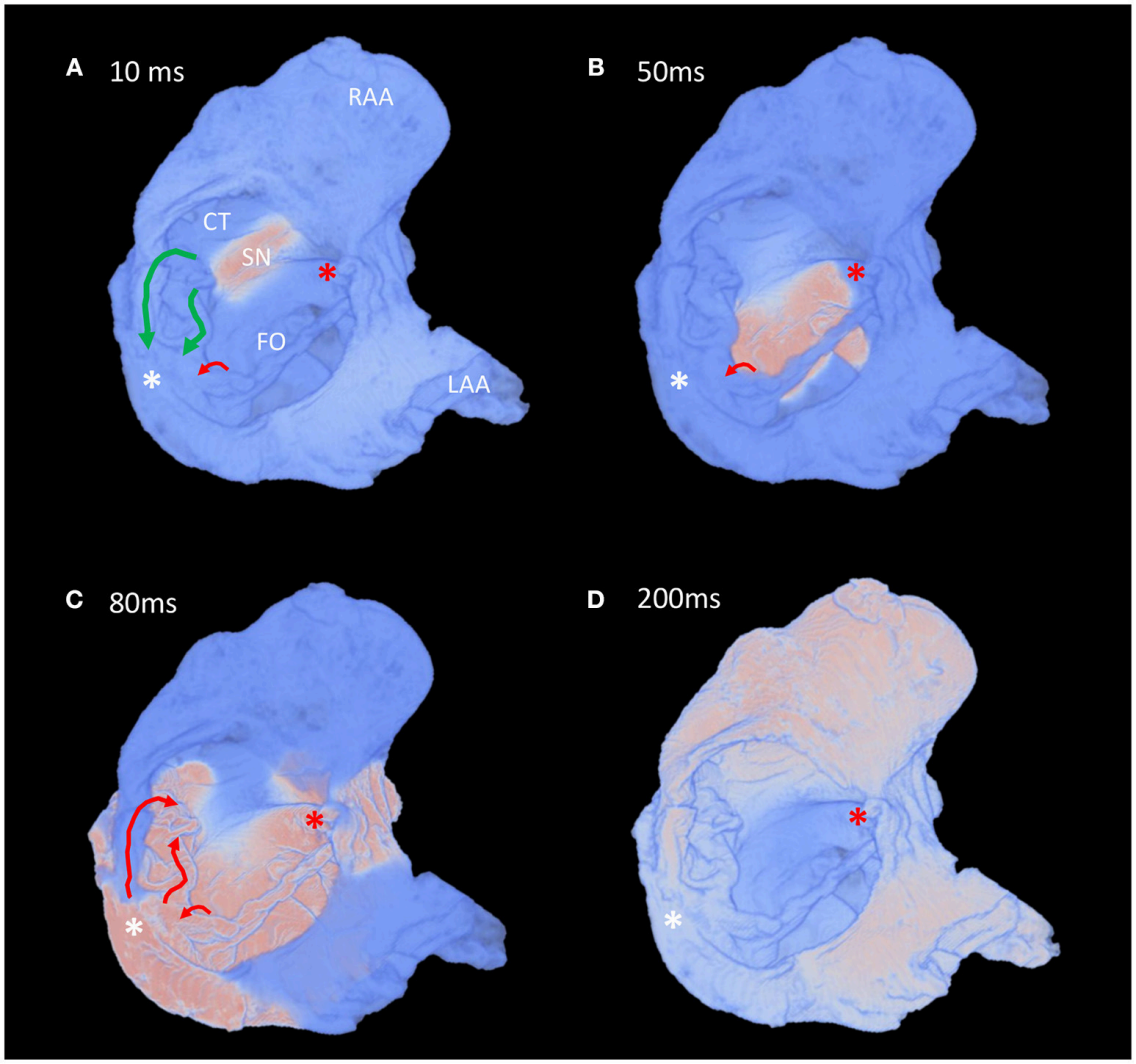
Fast pacing elicits retrograde conduction via the “slow” pathway in the atria of a heart with AVSD. (A–D) Time-lapse snapshots taken from the Supplementary Video 3 showing preferential inter-nodal conduction via the region normally associated with the “fast” pathway, and subsequent retrograde conduction up the “slow” pathway, during an atrial pacing protocol (s1-s2 interval 300 ms). Views are comparable to those presented in Figure 7. The normal location and direction of the “slow” pathway (green), and the septal aspect of the elongated “fast” pathway (red) are indicated by solid arrows. Pink indicates activated myocardium, light blue indicates dormant myocardium. See methods for modeling parameters. White*, location of compact atrioventricular node in AVSD heart; Red*, location of compact atrioventricular node in normal heart; CS, coronary sinus, CT, terminal crest; FO, fossa ovale; LAA, left atrial appendage; RAA, right atrial appendage; SN, sinus node.
